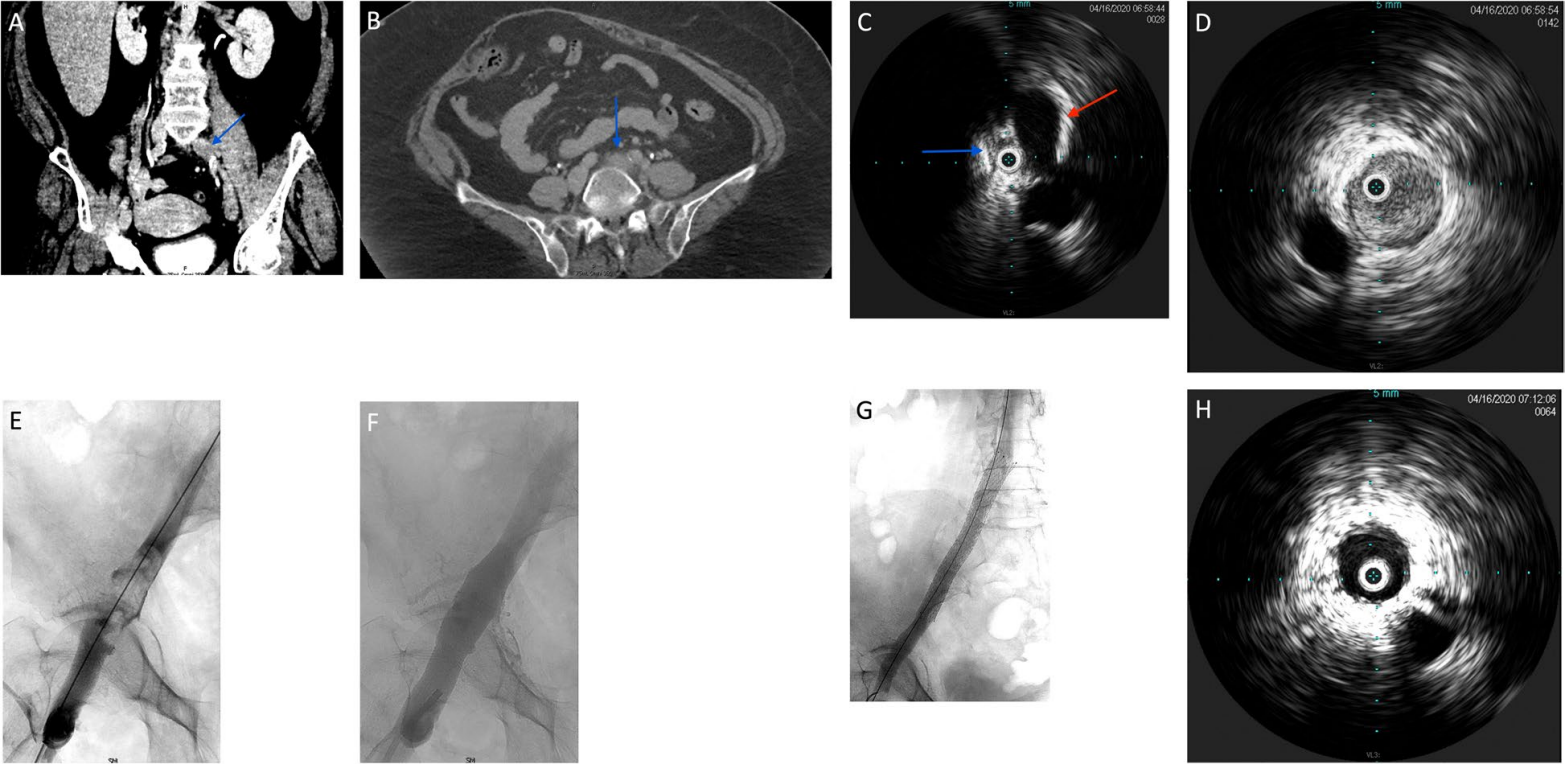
63-year-old woman presenting with acute left lower extremity swelling and pain. A Coronal contrast-enhanced CT demonstrates acute, completely occlusive thrombus extending from the proximal left common iliac vein (blue arrow) through the common femoral vein. B Axial contrast-enhanced CT at the level of the proximal left common iliac vein demonstrates fat-stranding (blue arrow) and expansile thrombus, suggestive of acute deep venous thrombosis. C Intra-vascular ultrasound of the left common iliac vein (blue arrow) demonstrates completely occlusive thrombus and marked luminal narrowing secondary to compression from the crossing right common iliac artery (red arrow), compatible with a May-Thurner compression lesion. D Pre-intervention IVUS of the left common iliac vein demonstrates completely occlusive, expansile acute thrombus. E Venography of the left iliofemoral veins demonstrate multiple filling defects compatible with acute thrombus. F Venography following treatment with the ZelanteDVT system demonstrates technically successful thrombectomy with no residual thrombus. G Completion venography following stent placement demonstrates resolution of the compressive lesion at the left common iliac vein and brisk in-line venous flow through the iliofemoral veins without evidence of residual thrombus. H Completion IVUS following stent placement demonstrates complete restoration of luminal size without residual thrombus or narrowing
